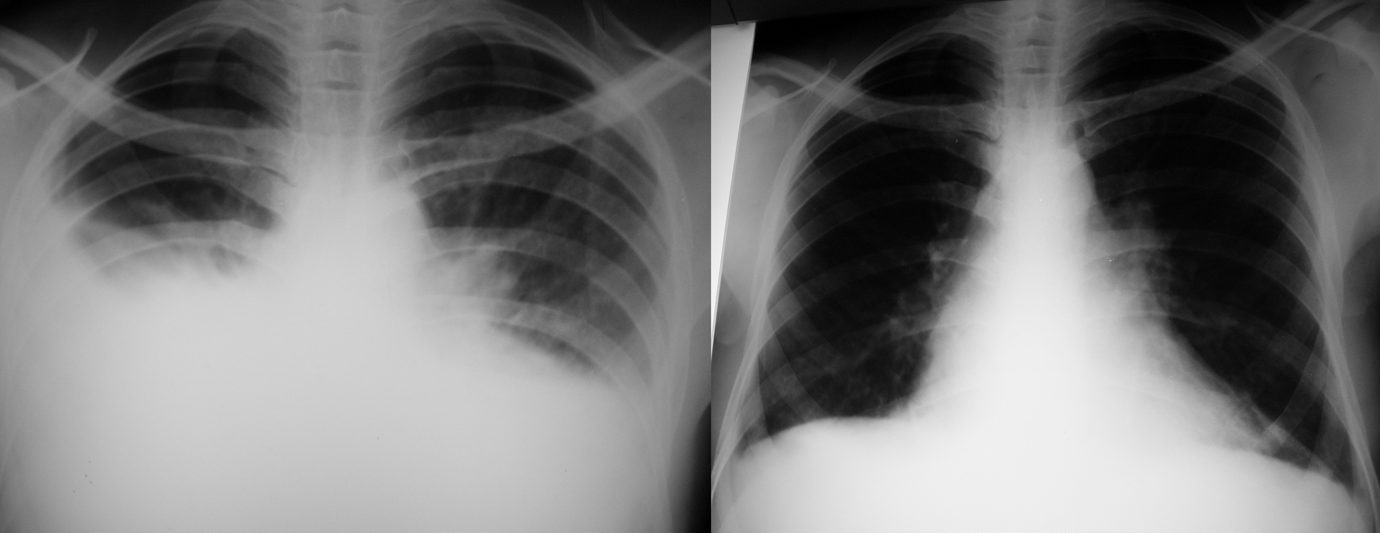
Resolution of pleural effusions after treatment for loiasis. Chest radiographs showing bilateral basilar densities at the time of admission (left) and complete resolution at 3-week follow-up after one dose of ivermectin (right).
